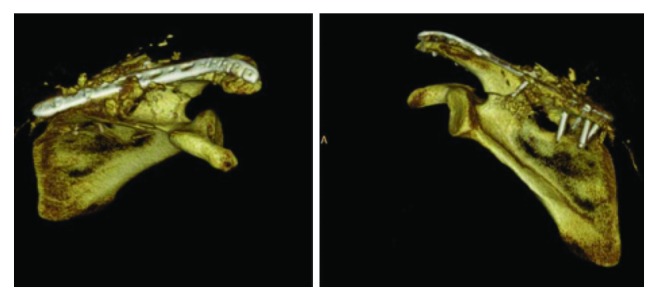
Postoperative computed tomography 3-dimensional reconstruction of the scapula showing our plate fixation of the scapular spine fracture.
